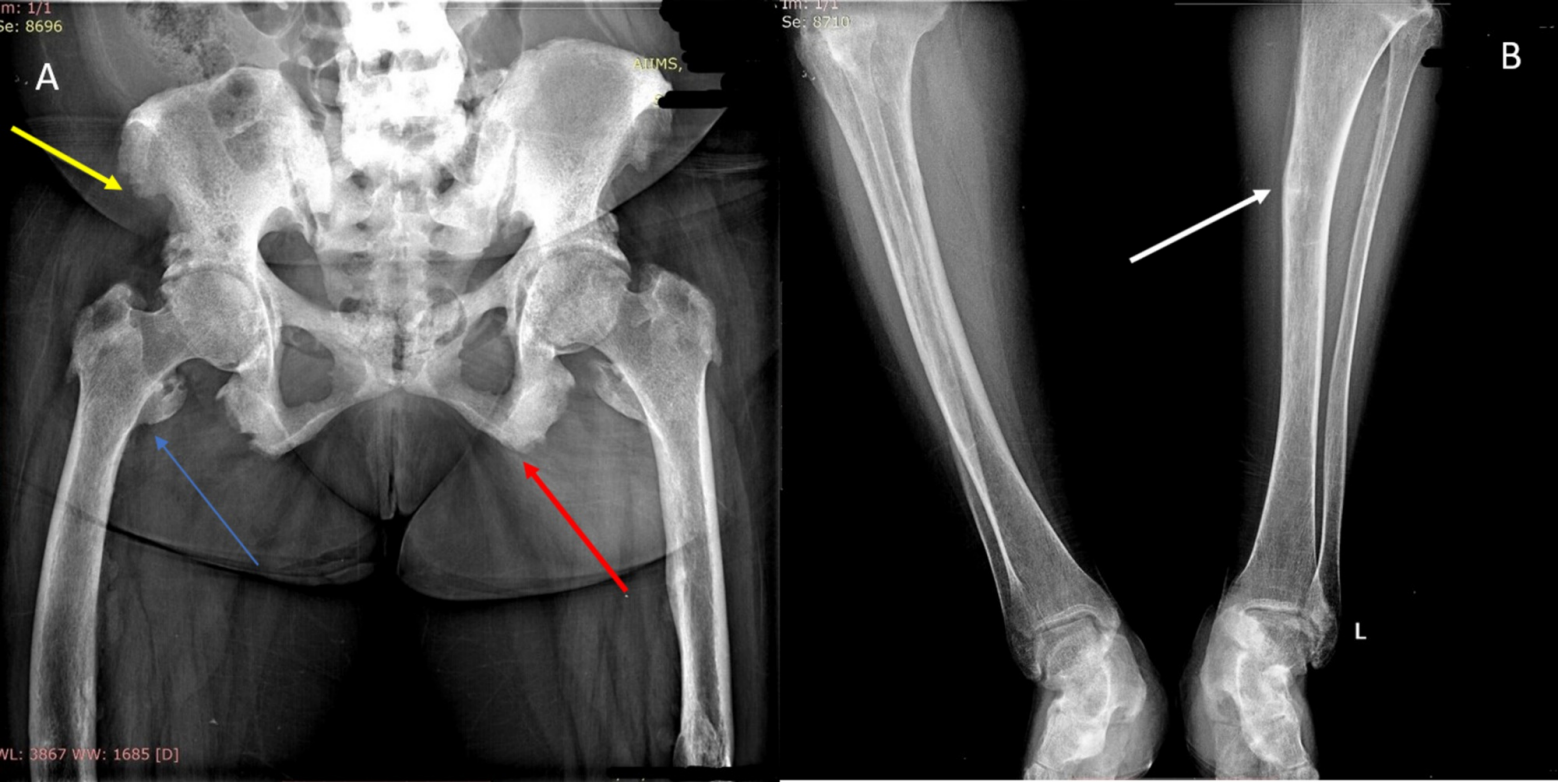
Image 1A showing enthesopathy and 1B showing osteopenia and insufficiency fracture 1A: pelvic radiograph anteroposterior view with proximal thighs showing irregularity at bilateral anterior superior iliac spine (yellow arrow), at the bilateral ischial tuberosity (red arrow), and at greater and lesser trochanters (blue arrow) suggestive of enthesopathy. Bilateral axial migration of femoral heads is present, suggesting protrusio acetabuli. 1B: bilateral leg radiograph showing osteopenic tibia and fibula along with insufficiency fracture at left tibial diaphysis (white arrow)
